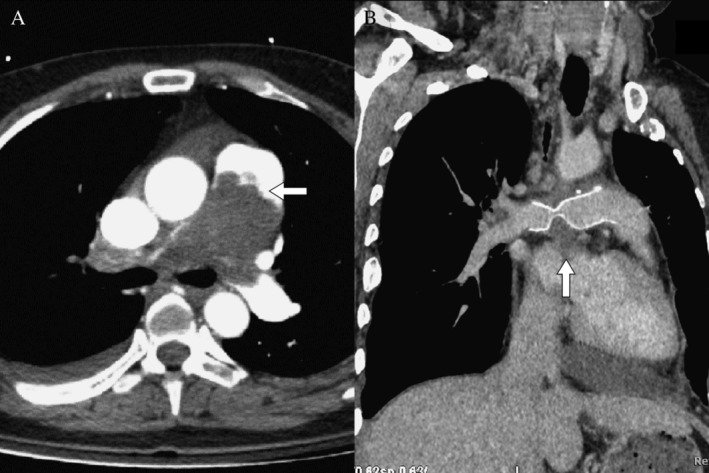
(A) Axial view of computed tomography (CT) angiography showing soft tissue‐like filling defects in the pulmonary trunk and extending into the left and right pulmonary arteries; the proximal edge of the mass was nodular structure (arrow) and the distal part of lesion was dilated and had a grape‐like appearance. There was heterogeneous contrast enhancement. (B) Chest computed tomography (CT) with contrast after operation showed residual tumour (arrow) with external compression on graft.
